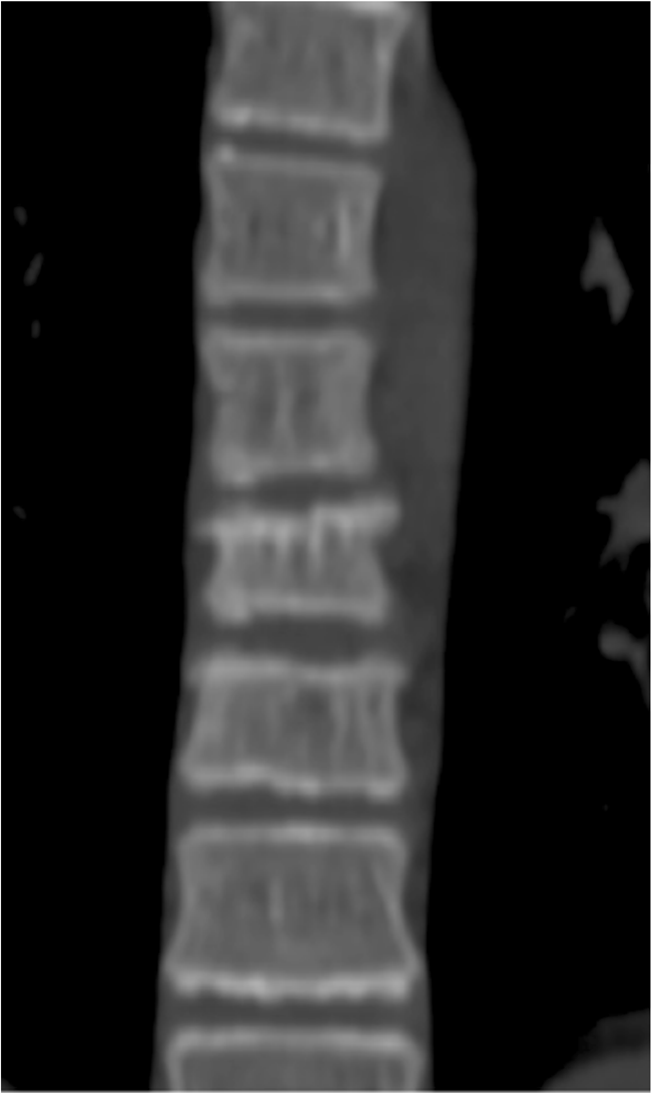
CT scan of fractured VB (coronal)
